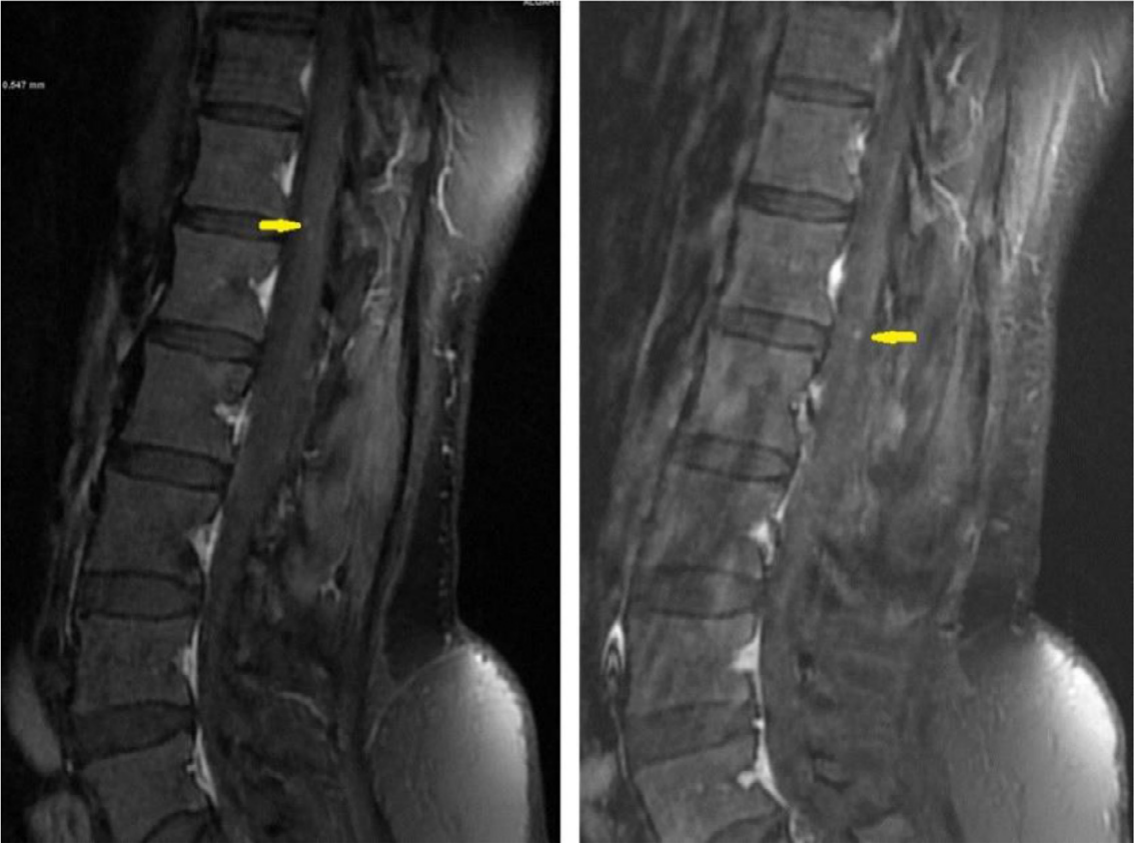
MRI lumbar spine 28 months after surgery show tiny enhancing nodules in the cauda equina consistent with CSF seeding (arrows).
